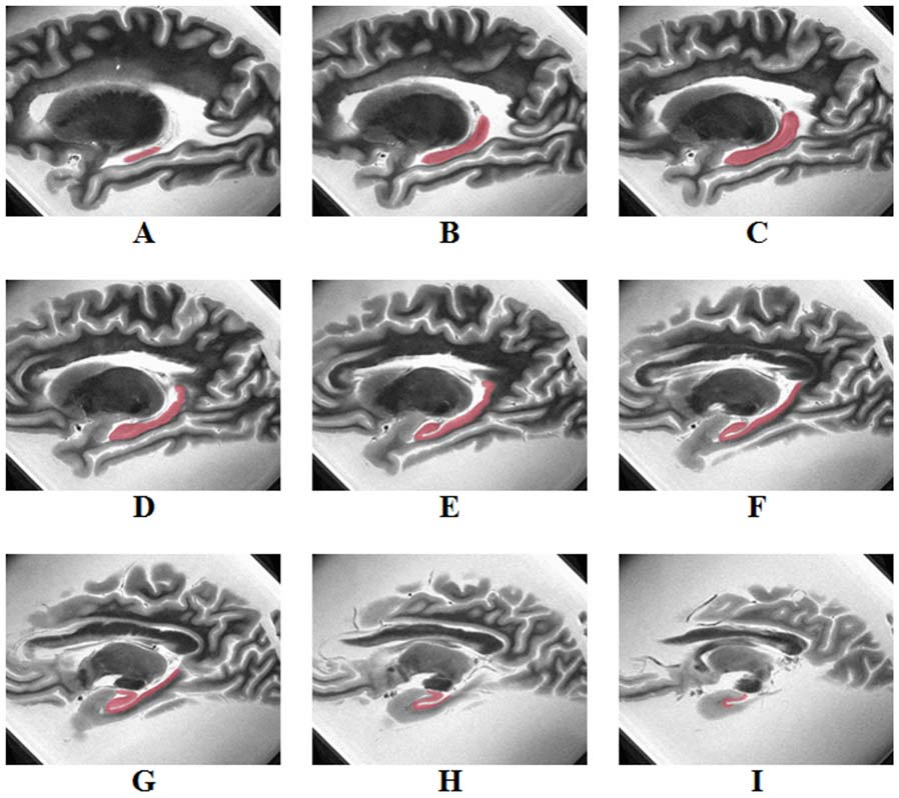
A typical three-dimensional region of interest created by manually outlining the hippocampal formation in consecutive T_2_-weighted sagittal images of a human cerebral hemisphere. Image A corresponds to the most lateral slice and image I to the most medial slice. The hemisphere remained immersed in formaldehyde solution during the MRI scan. In-plane resolution was 0.3125×0.3125 mm^2^ after zero-padding of the k-space acquisition matrix.
